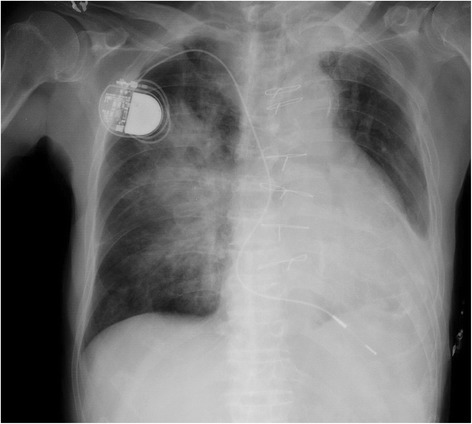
Portable chest radiograph the next day in the ICU. The patient was in the supine position. Diffuse hilar haziness remained. The right hemidiaphragm had moved to the sixth intercostal space, suggesting restoration of right hemidiaphragmatic function
